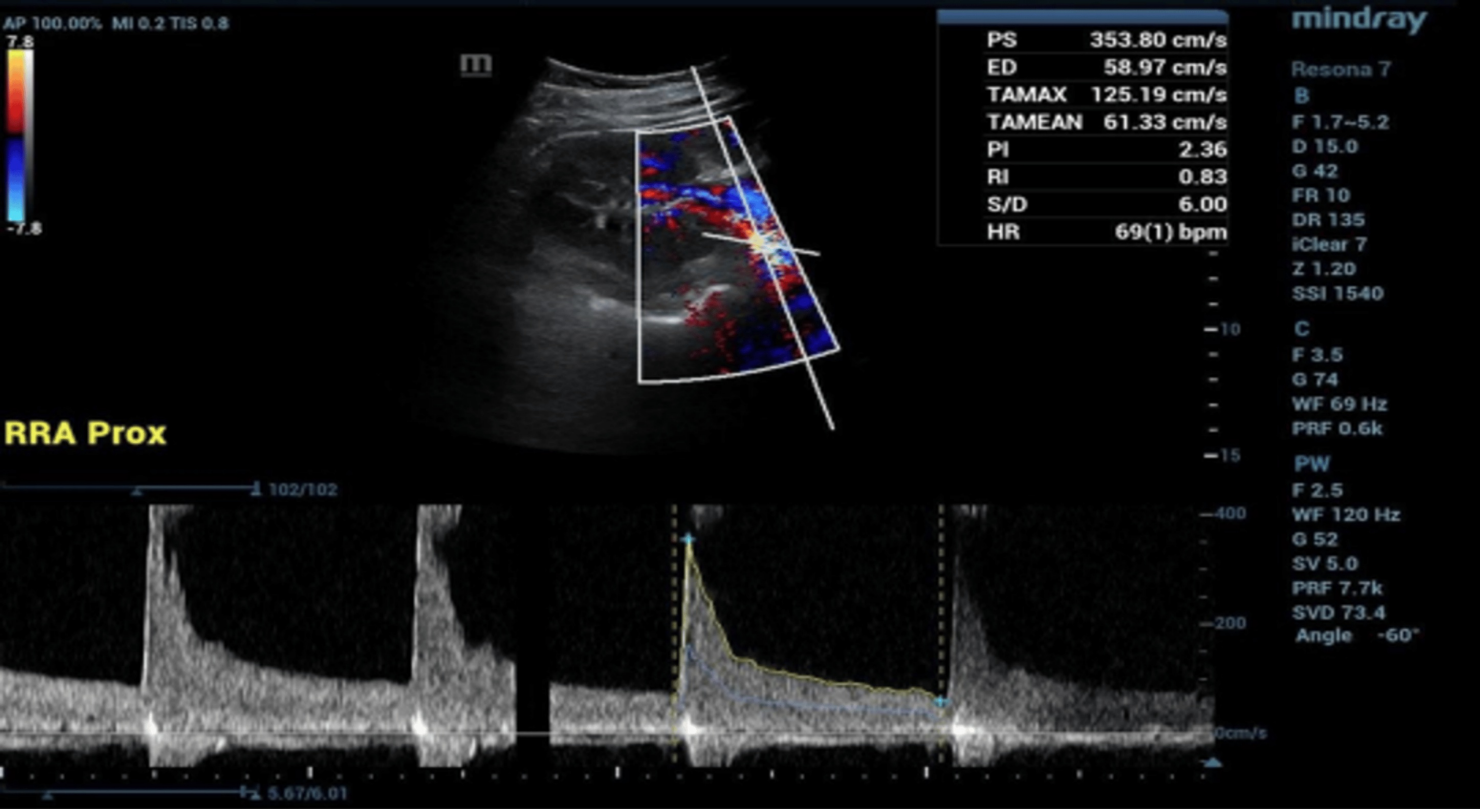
Right renal artery vascular duplex ultrasound Peak systolic velocities were 353.8 cm/s proximally, 330 cm/s in the mid-right renal artery, and 291.4 cm/s distally. These measurements suggest stenosis of the right renal artery greater than 60%. The renal resistive index was 0.7. The renal-aortic ratio was 3.1.
